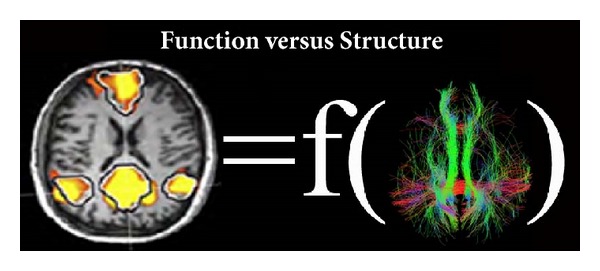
Default mode network in a healthy control as extracted from resting state functional magnetic resonance imaging using independent component analysis and the fibers reconstructed using a tractography technique applied to diffusion tensor imaging data and subsequently filtered by the regions functionally connected in the default mode network.
